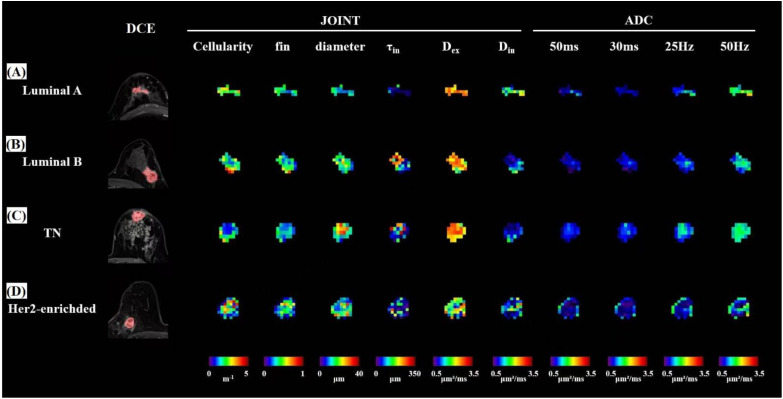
Diffusivity MRI maps in four participants with different molecular subtypes using pulsed gradient spin-echo (PGSE) and oscillating gradient spin-echo (OGSE) sequences, including cellularity, intracellular volume fraction (f_in_), cell diameter, intracellular water residence time (τ_in_), extracellular and intracellular diffusivity (D_ex_, D_in_), and the four ADC maps (ADC_50ms_, ADC_30ms_, ADC_25Hz_, and ADC_50Hz_). The bottom of figure shows the corresponding specific values. (A) A 45-year-old female with Luminal A breast cancer; (B) a 50-year-old female with Luminal B breast cancer; (C) a 49-year-old female with triple-negative (TN) breast cancer; (D) a 39-year-old female with HER2-enriched breast cancer.
